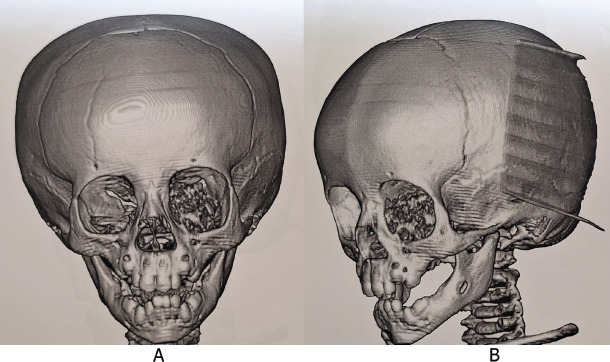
Computed tomography (CT) scan of the skull with 3D reconstruction. (a): Front view. (b): Lateral view. An image of irregularly oval morphology of defined contours is observed, which is located in the region of the left orbit showing involvement of the left ethmoid orbital lamina and the greater and lesser wing of the left sphenoid with moth-eaten pattern, chondroid matrix, showing disruption of the cortical, with a solid portion and also displacement of the eyeball with approximate dimensions of 33 × 31 mm. Source: Medical registry.
